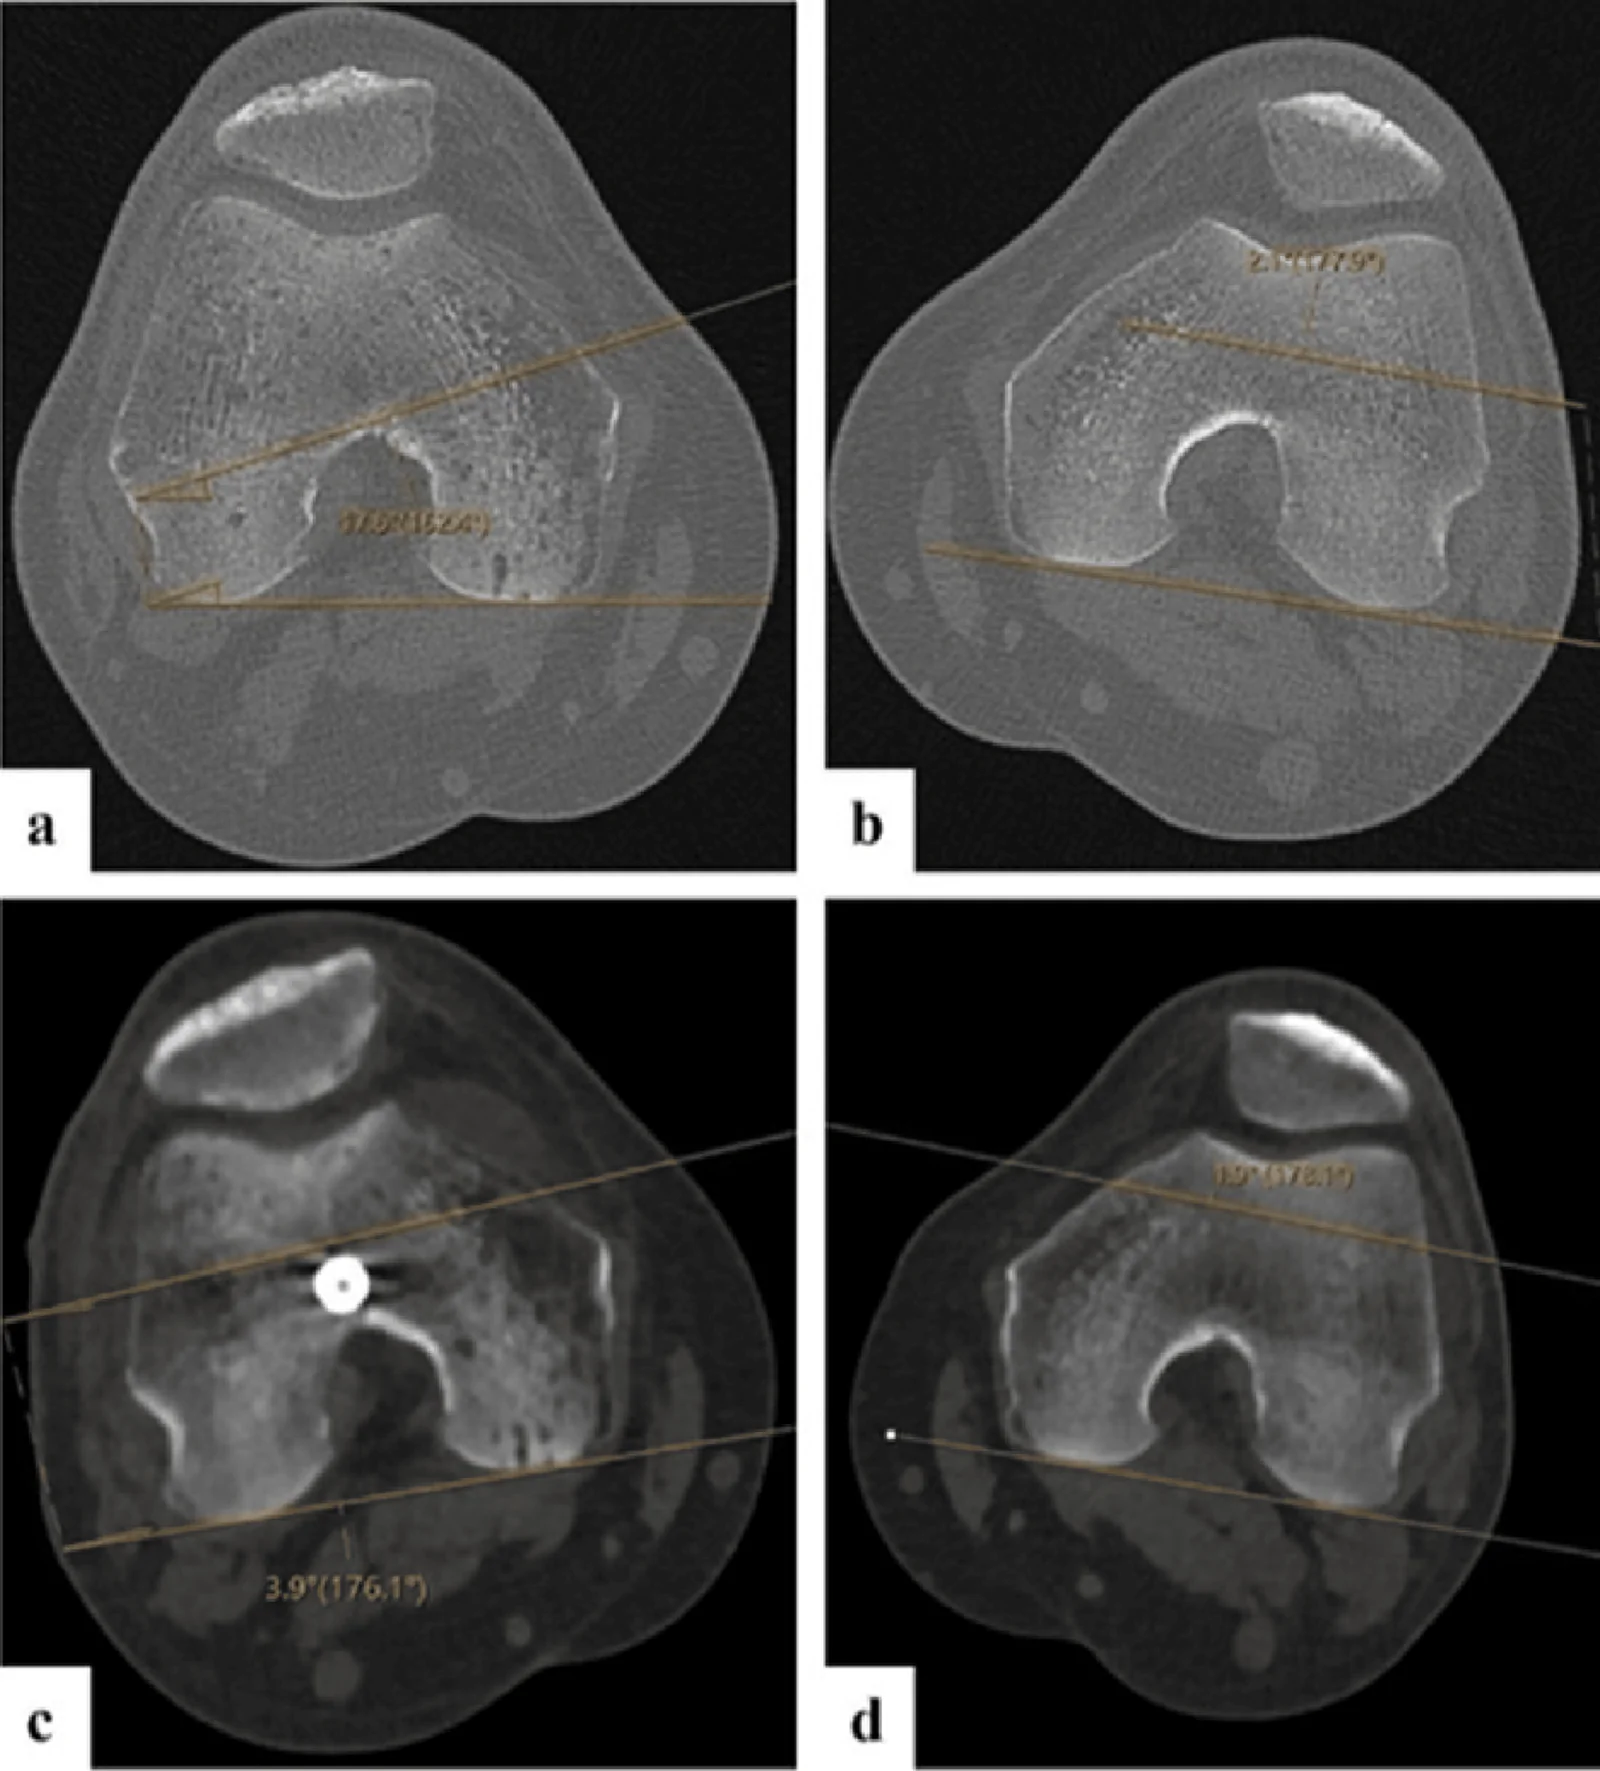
Computed Tomography Scanogram Non-contrast CT was used to determine femoral anteversion of both affected and unaffected lower extremities. a) Preoperative CT of the affected extremity shows a femoral anteversion of 17.6° b) Preoperative CT of the unaffected extremity shows a femoral anteversion of 2.1° c) Postoperative CT of the affected extremity shows a femoral anteversion of 3.9° d) Postoperative CT of the unaffected extremity showing a femoral anteversion of 1.9°.
